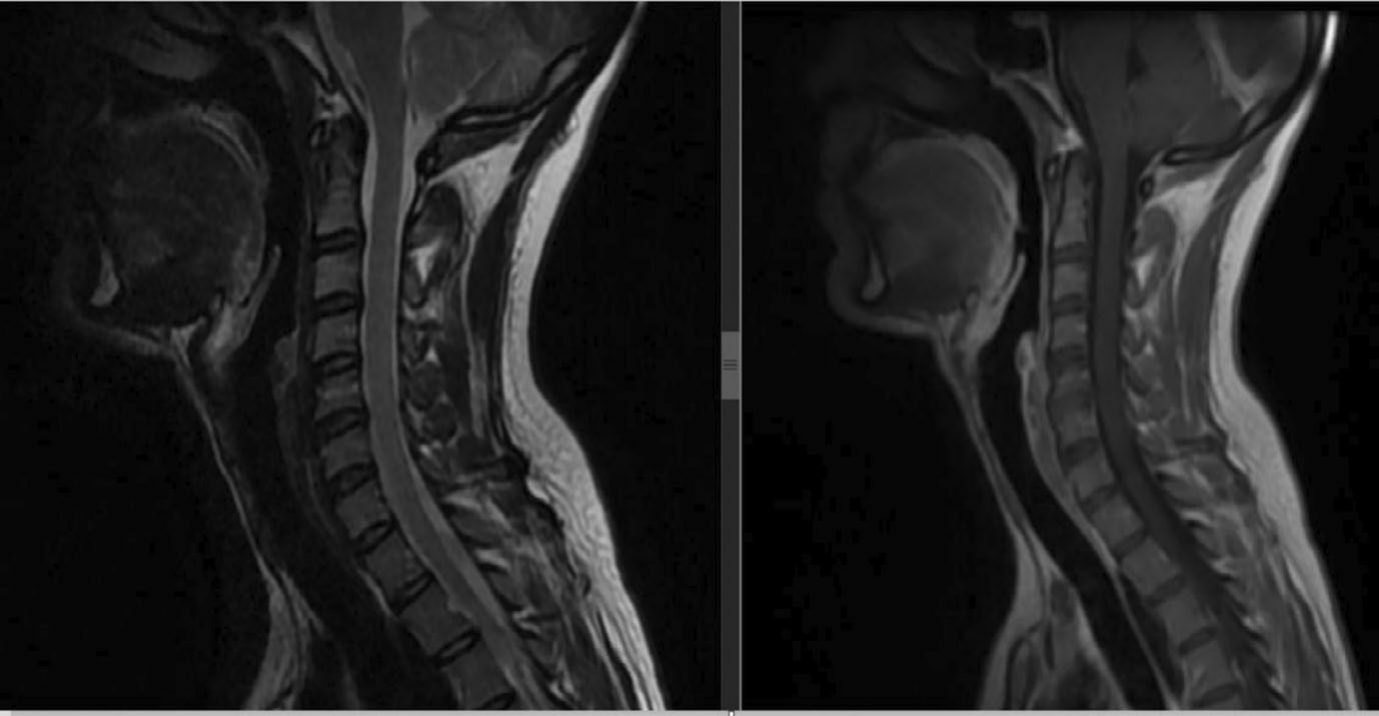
T1 weighted (right) and T2 weighted (left) cervical MRI sagittal view (normal). MRI, magnetic resonance imaging.
